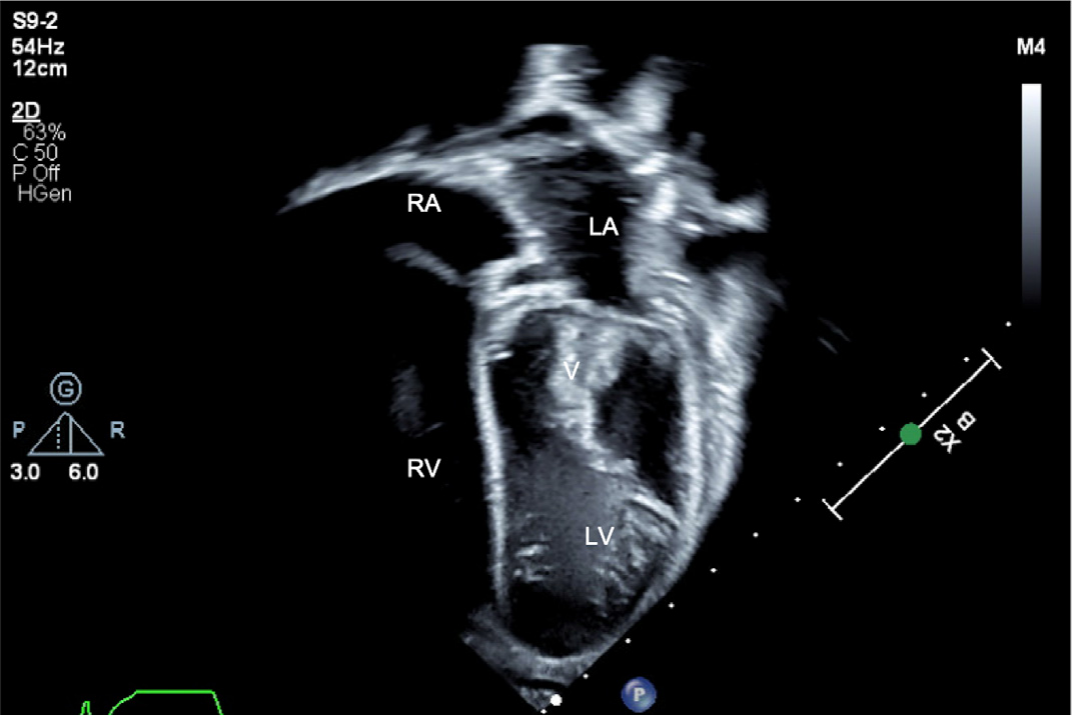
Echocardiogram of Vegetations: Mitral Valve Transthoracic apical 4-chamber view demonstrating a 24 × 10-mm vegetation on the mitral valve. LA = left atrium; LV = left ventricle; RA = right atrium; RV = right ventricle; V = vegetation.
